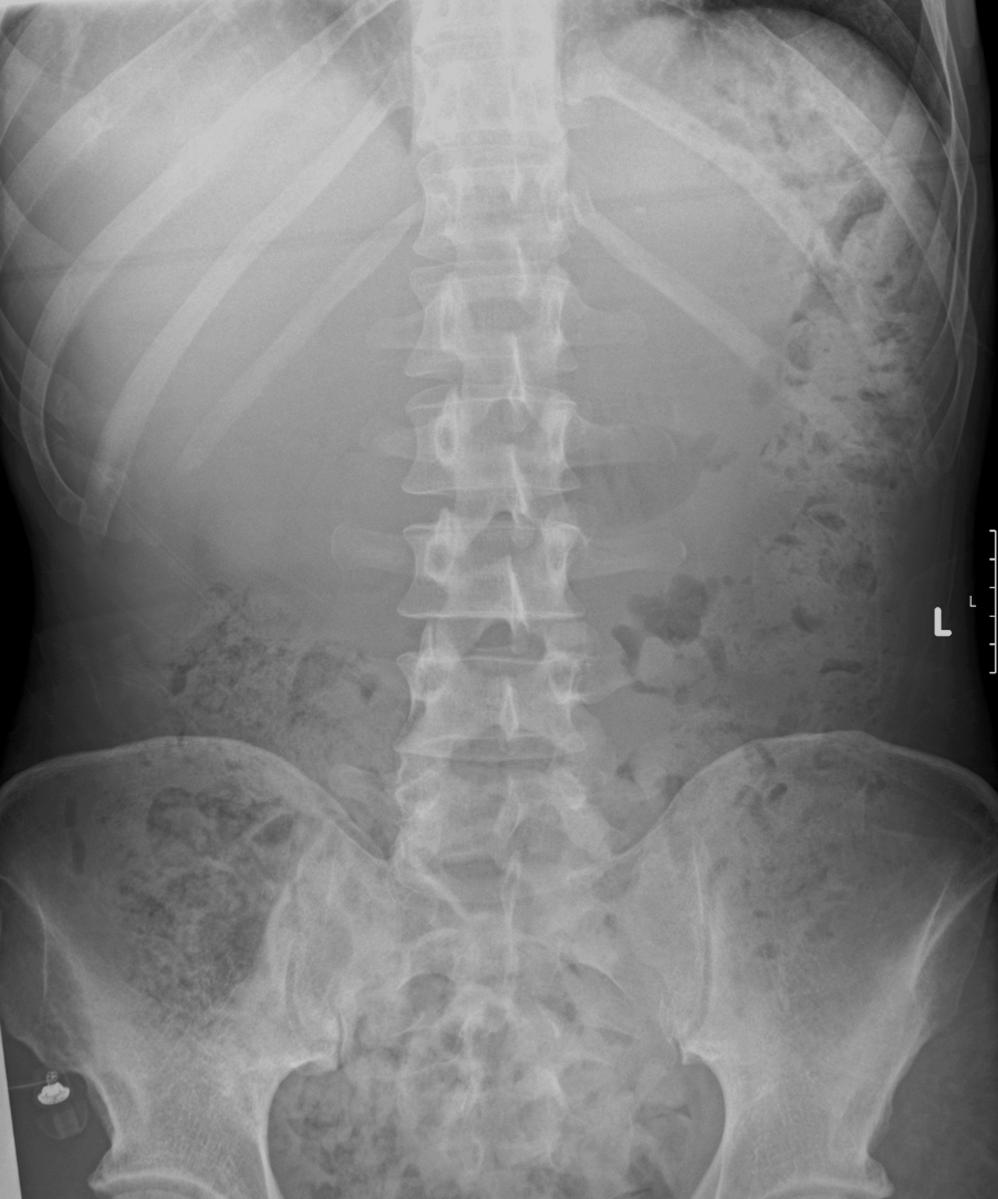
Abdominal X-ray (plain) Findings: There is a 2 mm calcified opacity projected over the left kidney shadow - possible left kidney stone. Moderate faecal loading noted. Opacities in the pelvic cavity are most likely phleboliths.
